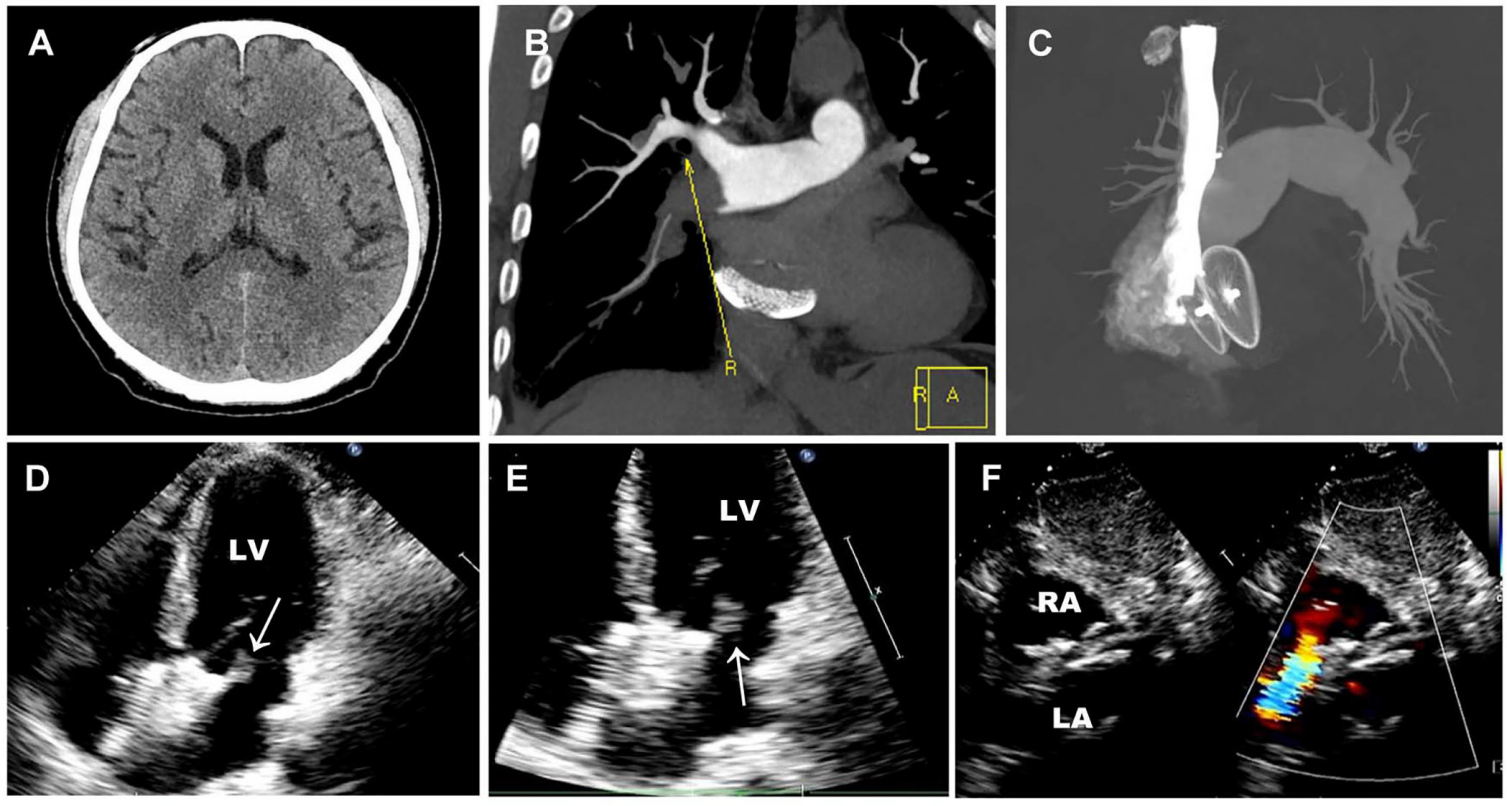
Preoperative imaging examination. (A) Brain CT manifested as post-infraction of cerebral lacunar with no other significant abnormalities. (B,C) Pulmonary CT angiography presented embolism in the distal of the right pulmonary artery and its branches. (D–F) Preoperative TTE showed a 13 × 9 mm thrombus floating in the left atrium without a shunt. White arrows represent thrombus.
